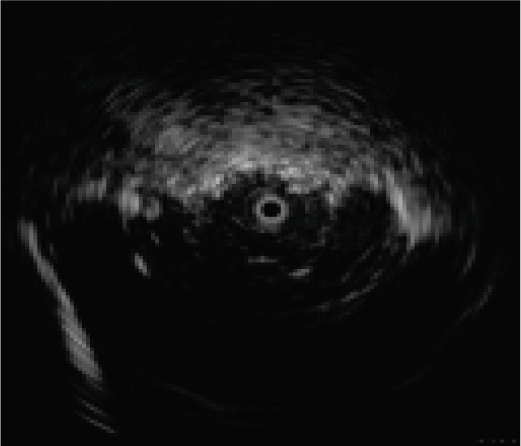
Radial probe endobronchial ultrasound (RP‐EBUS) image of the left upper lobe reveals abnormal echoes corresponding to the lesion.
